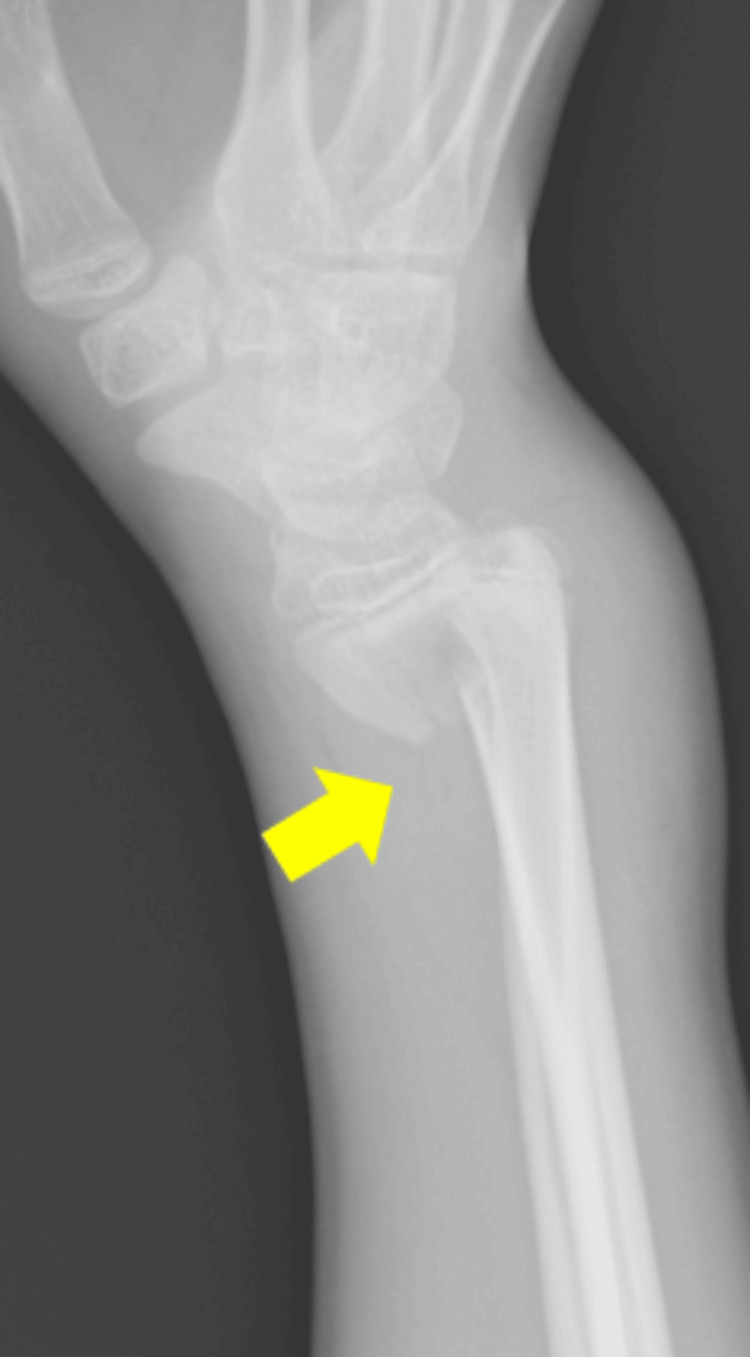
X-ray at the time of injury (yellow arrow)
